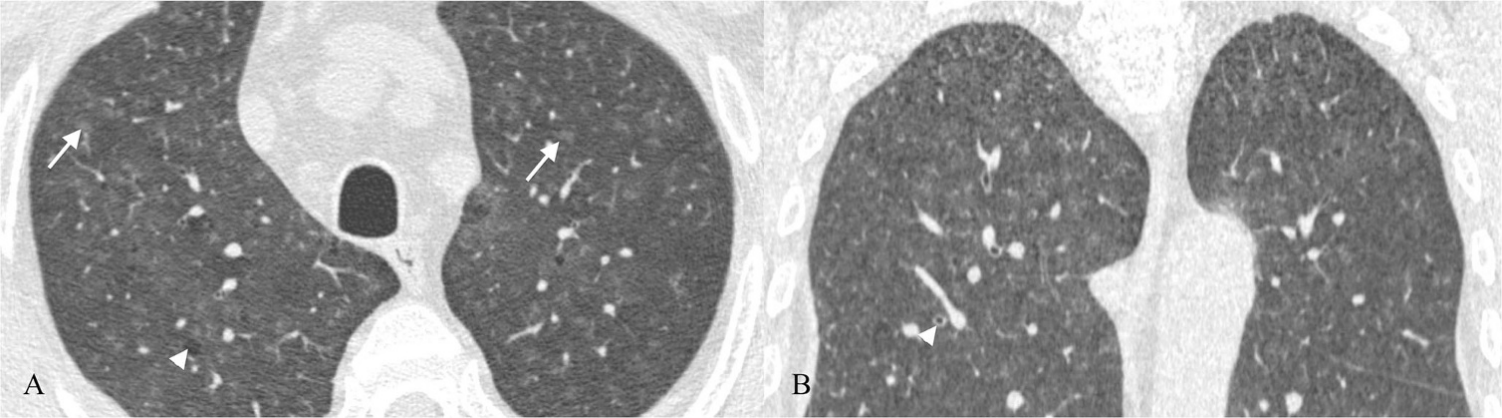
RB-ILD pattern in a 52-year-old smoker patient. Poorly defined centrilobular nodules (white arrows), due to peribronchial extension of the infiltrate, are uniformly disseminated through the upper lobes. Other CT features very frequently encountered are centrilobular emphysema (white arrowhead)
